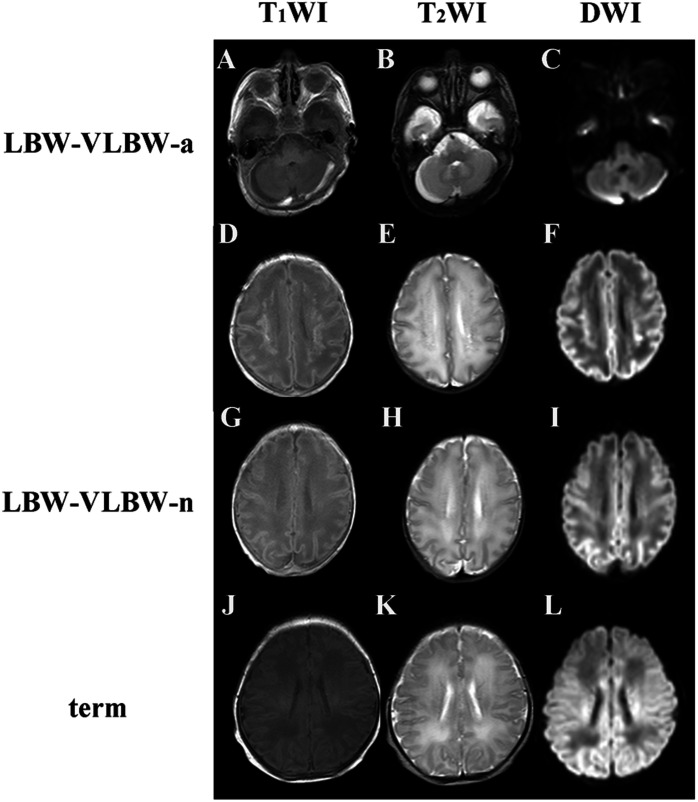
T1-, T2-, and diffusion-weighted imaging (T1WI, T2WI, and DWI) studies of low birth-weight/very low birth-weight (LBW–VLBW) and term neonates: (A–C) 35^+1^ week-old male newborn (birth weight, 2,000 g) with subarachnoid hemorrhages of posterior cranial fossa (LBW–VLBW-a subset); (D–F) 35^+1^ week-old female newborn (birth weight, 2,200 g) with white matter damage and periventricular leukomalacia (LBW–VLBW-a subset), showing multiple increased signal intensities on T1WI and DWI, decreased signal intensity on T2WI, and cystic lesions of centrum semiovale bilaterally; (G–I) 34^+5^ week-old male newborn (birth weight, 1,625 g) with no MRI abnormalities (LBW–VLBW-n subset); and (J–L) maps indicating 42^+3^ week-old male term newborn (birth weight, 3,400 g) with no MRI abnormalities.
